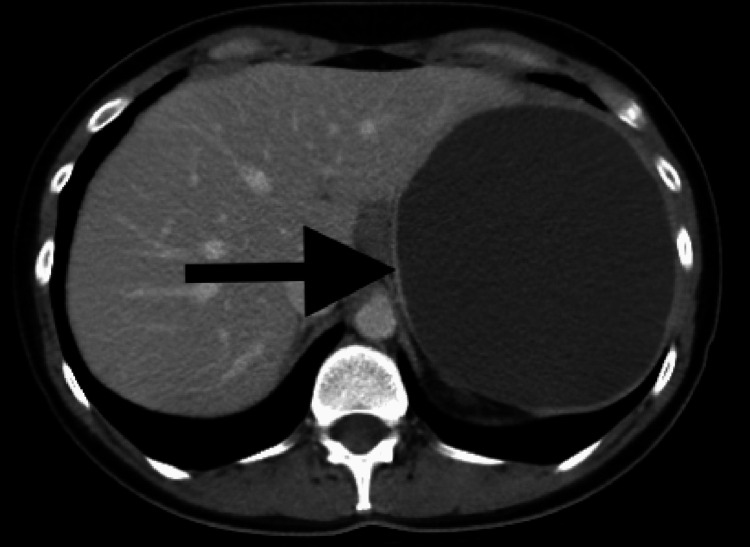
Computerized tomography (CT) of the abdomen/pelvis with intravenous contrast: a large cyst seen in the spleen measuring 11.9 × 10.6 cm in the axial dimension. Mass effect on the stomach can be seen with the partial displacement of the pancreas and kidney.
